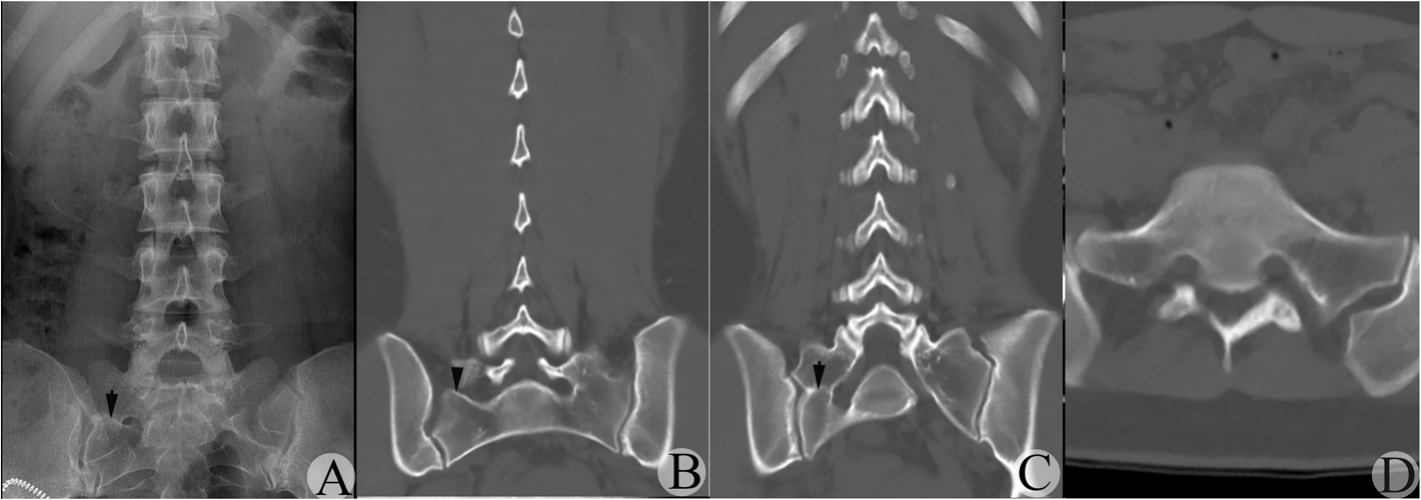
A suspected type IV MA-LSTV judged by AP-LPR was verified to be type IIIb by CT-CRI. a AP-LPR image showing the suspected JLS existed on the right side with narrowed connection width and RSB. Arrow indicates the suspected JLS. b CT-CRI showing the right TP of the MA-LSTV separated from the sacrum in some CT-CRIs through the vertebral arch planes. Arrowhead indicates the JLS with vague RSB on the right side. c CT-CRI showing BUS on the right side with RSB. Arrow indicates the BUS with RSB. d Transverse CT image confirmed bony connection bilaterally, while the connection region was much narrower and shorter on the right side compared to the left side
